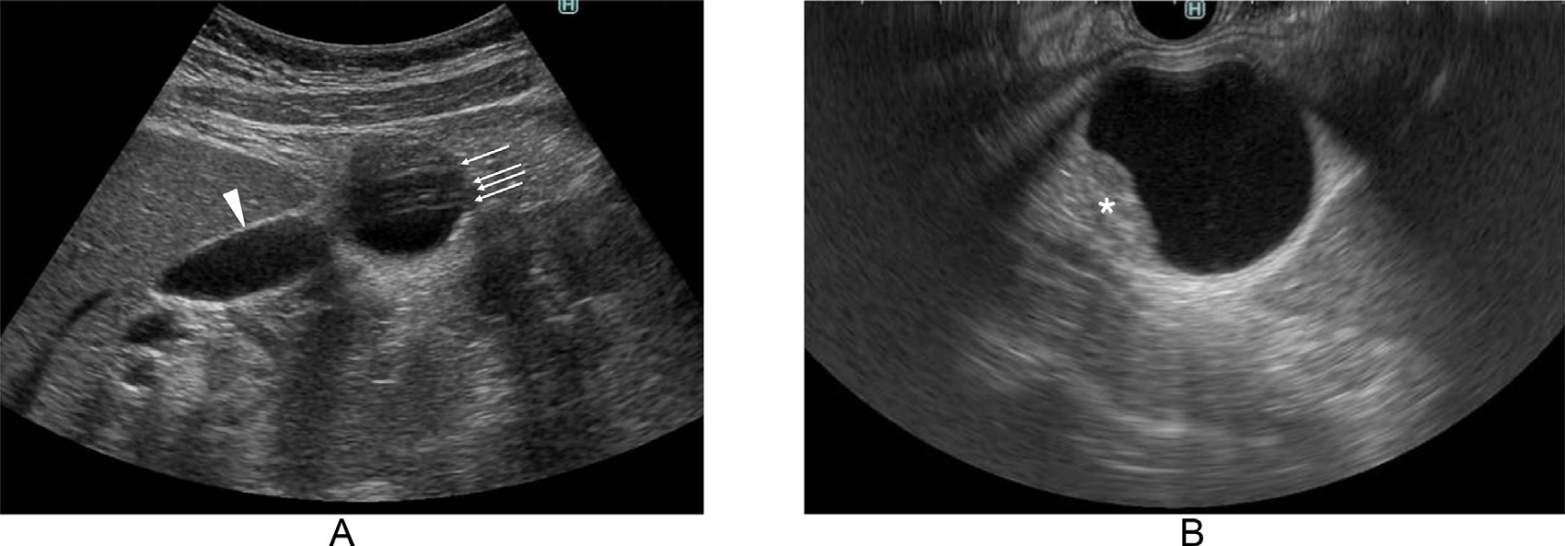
Ultrasound findings. (A) Ultrasound could not negate the continuity between the gallbladder (arrowhead) and the cystic lesion containing the obscured solid part. The presence of multiple reflections of ultrasound (arrows) further made it difficult to evaluate the continuity between the 2. (B) Endoscopic ultrasound clearly showed the intra-cystic solid tumor (asterisk).
